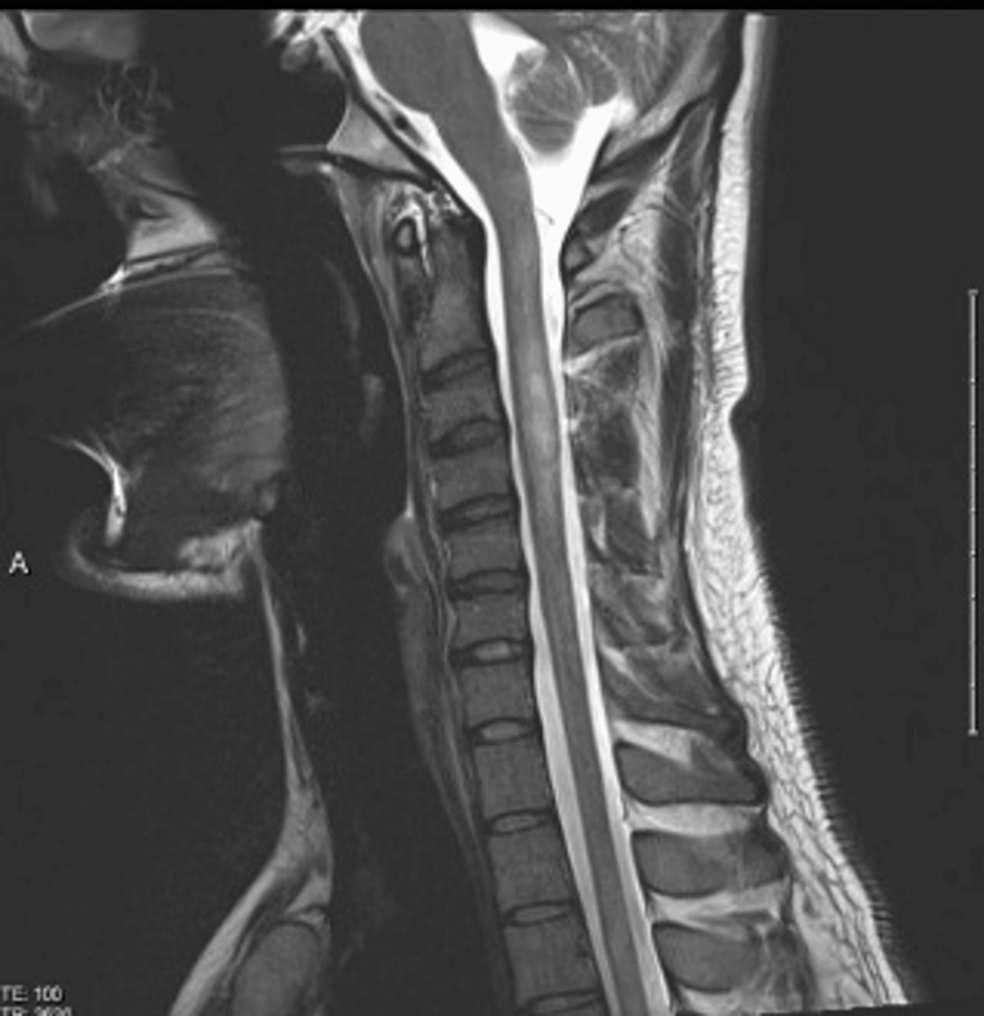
MRI of the cervical spine T2 with contrast. It shows hyperintense signal intensity on T2 image with enhancement post gadolinium.
